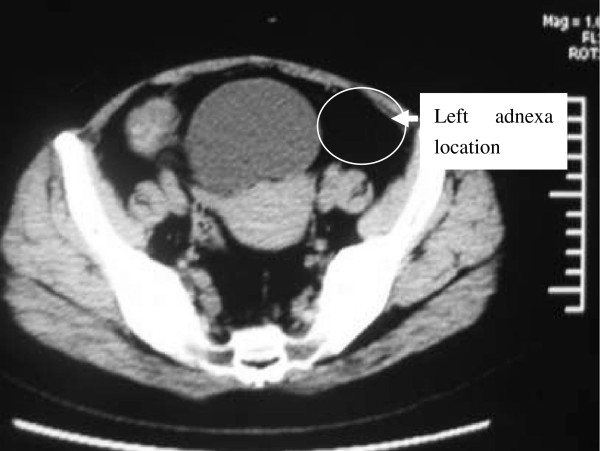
Pelvic CT scan showing no recurrent or residual mass in the left adnexa location, and the right ovary and the uterus were of normal appearance.
